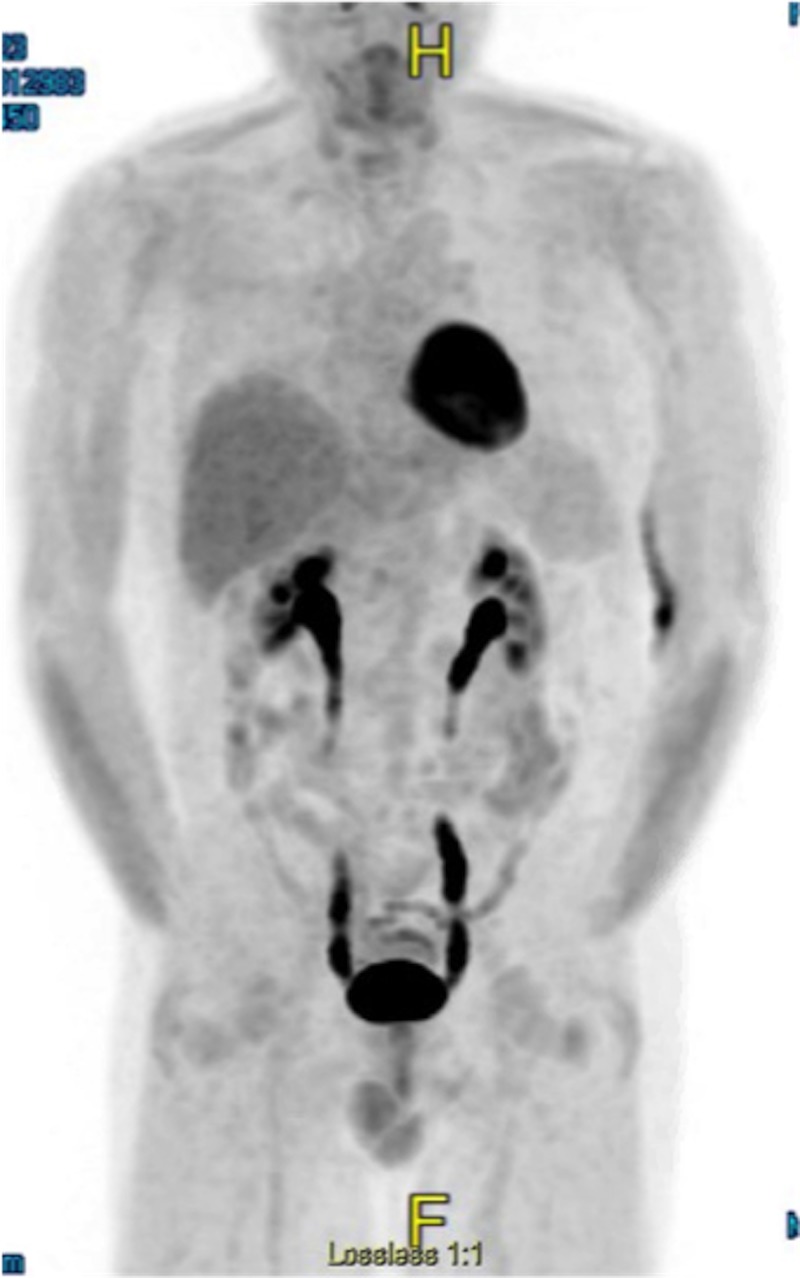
Post-treatment PET/CT PET/CT scan after treatment showing resolution of previously seen lymphadenopathy. PET/CT: positron emission tomography/computed tomography
